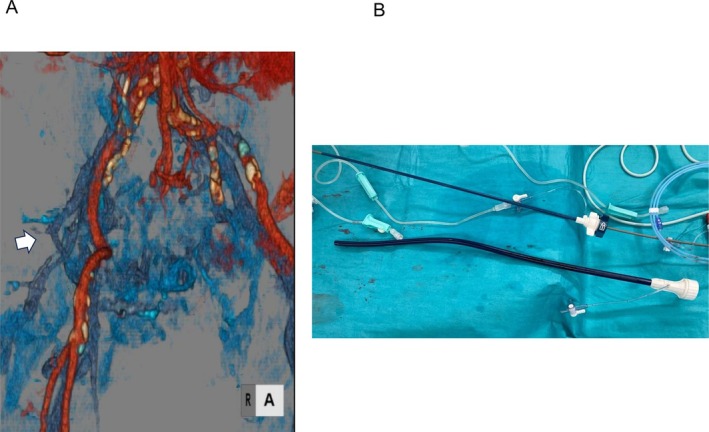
(A) Post‐procedural computed tomography (CT) obtained after two large‐bore sheath insertions demonstrated significant stenosis of the right common iliac vein (white arrow), corresponding to the venous narrowing that had been encountered intraoperatively. (B) The delivery sheath is shown after completion of the atrial leadless pacemaker implantation via the left femoral vein. Marked bending and curvature of the sheath are visible, reflecting the technical difficulty of the procedure.
